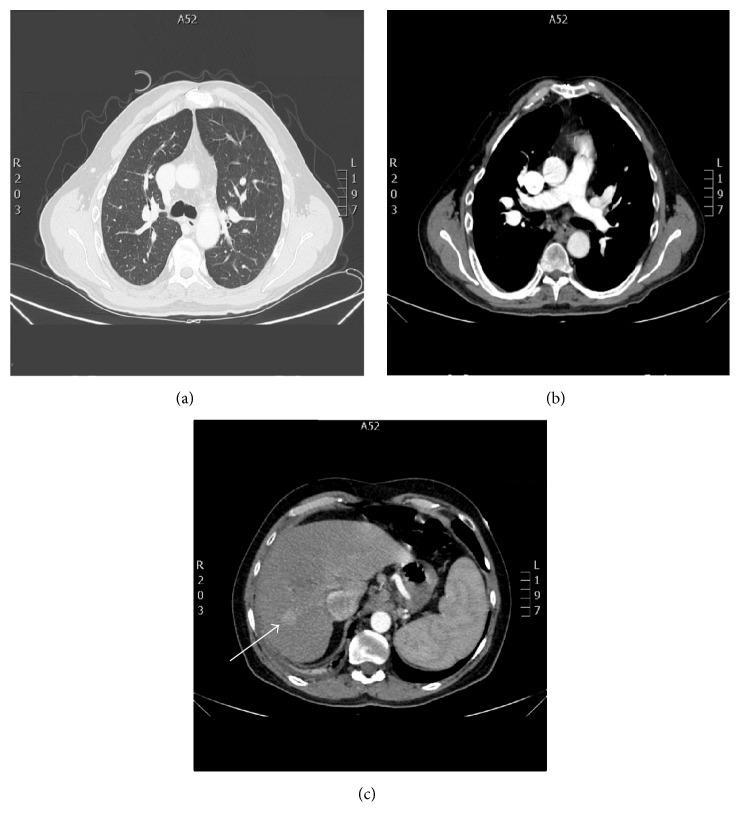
Parenchymal (a) and pulmonary vasculature study (b) on the chest CT scan showing no pathological findings; (c) abdomen CT scan showing a cirrhotic liver with a focal lesion (white arrow), highly suspicious of hepatocellular carcinoma.
